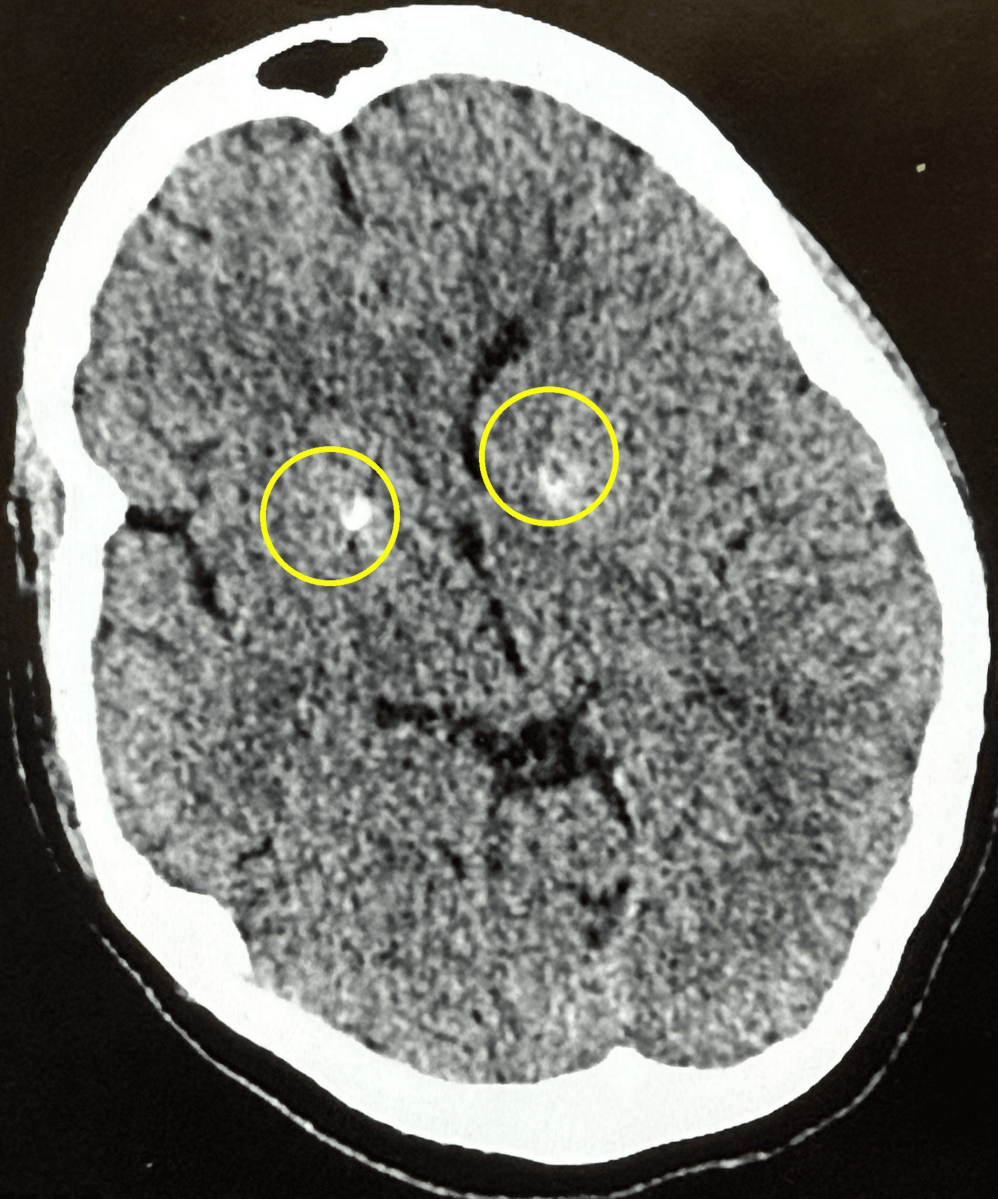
Non-contrast CT Brain Non-contrast CT scan of the brain demonstrating bilateral basal ganglia calcifications, indicated by yellow circles. No evidence of acute intracranial hemorrhage, mass effect, or space-occupying lesion is observed.
